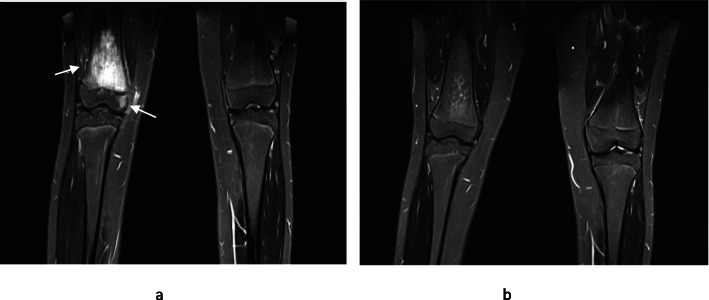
Pre and post pamidronate treatment MR images. Pre and post- pamidronate WB-MRI images of a 15 year old girl who presented with significant right knee pain and was diagnosed with CNO following a bone biopsy. Her symptoms resolved completely following four cycles of pamidronate. 2a – The coronal STIR MR image shows extensive high signal predominantly of the distal right femoral metaphysis consistent with intra-osseus oedema. A smaller area of the medial epiphysis is affected without features of cortical destruction or significant soft tissue component. 2b – Almost complete resolution of the metaphyseal high signal is in keeping with treatment response. The epiphyseal component is also no longer visible. In our exercise, the machine algorithm and panel of radiologists concurred that lesions resolved post treatment
